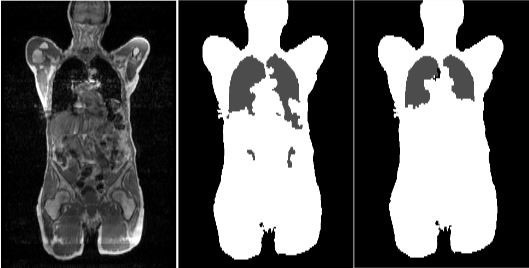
The magnetic resonance image (left), original segmentation (middle), and improved segmentation. Adapted from Hu et al.^69^
